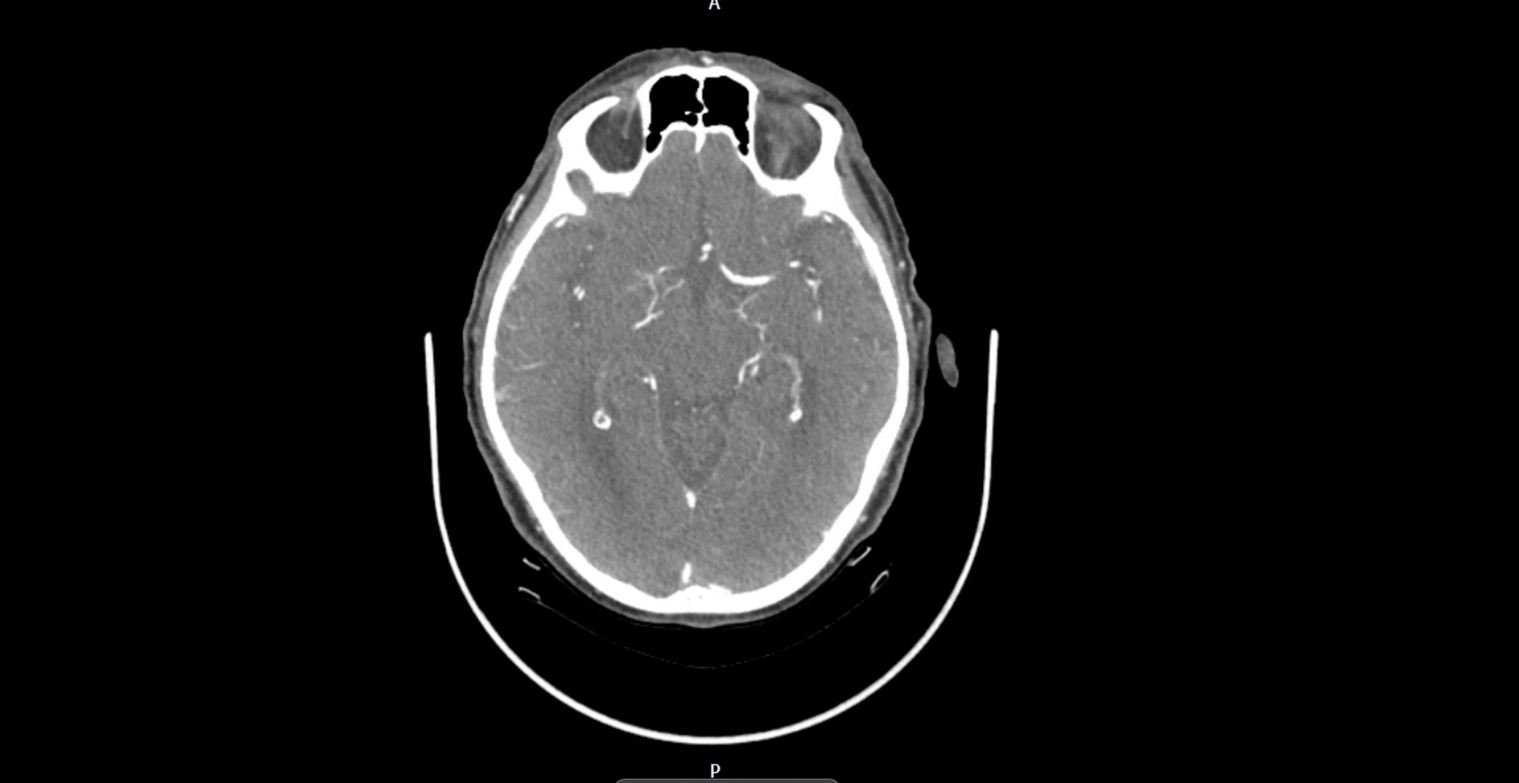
CT angiogram (intracranial, bilateral carotids, and aortic arch). Good opacification of the bilateral common carotid arteries. Mild to moderate narrowing of the bilateral carotid bulbs and proximal internal carotid arteries, with good opacification of the distal internal carotid arteries. Azygous anterior cerebral artery with good opacification of the distal segments. Good opacification of the bilateral middle cerebral arteries. Mildly hypoplastic left vertebral artery with a non-opacified distal intracranial segment. Good opacification of the right vertebral artery, basilar artery, and bilateral posterior cerebral arteries. No acute intracranial abnormalities detected; no evidence of thrombosis in the major intracranial arteries. CT, computed tomography
